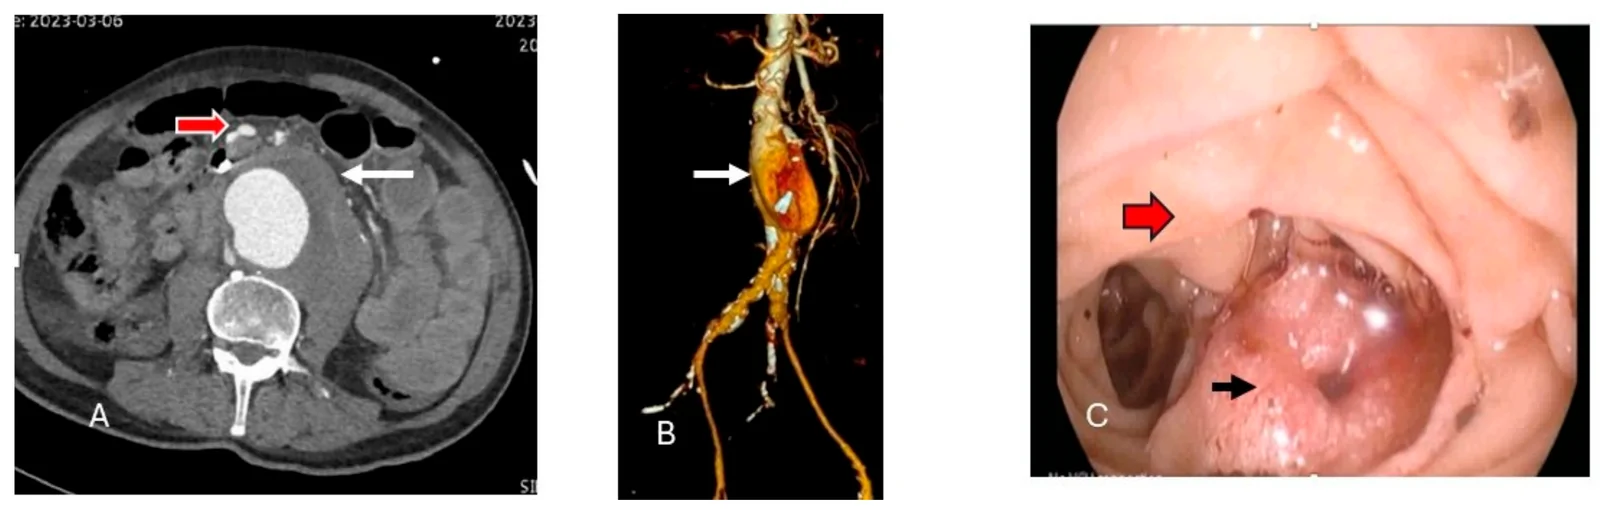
(Case 5): (A) CT scan with contrast (white arrow), indicating a large aneurysm with contrast in the small intestine (red arrow). (B) Reconstructed CT scan with contrast (white arrow), indicating the large infrarenal abdominal aortic aneurysm. (C) Endoscopy of the fourth part of the duodenum (red arrow) showing an ulcerating lesion with blood on the base of the ulcer (black arrow); it was actively bleeding during the endoscopy.
